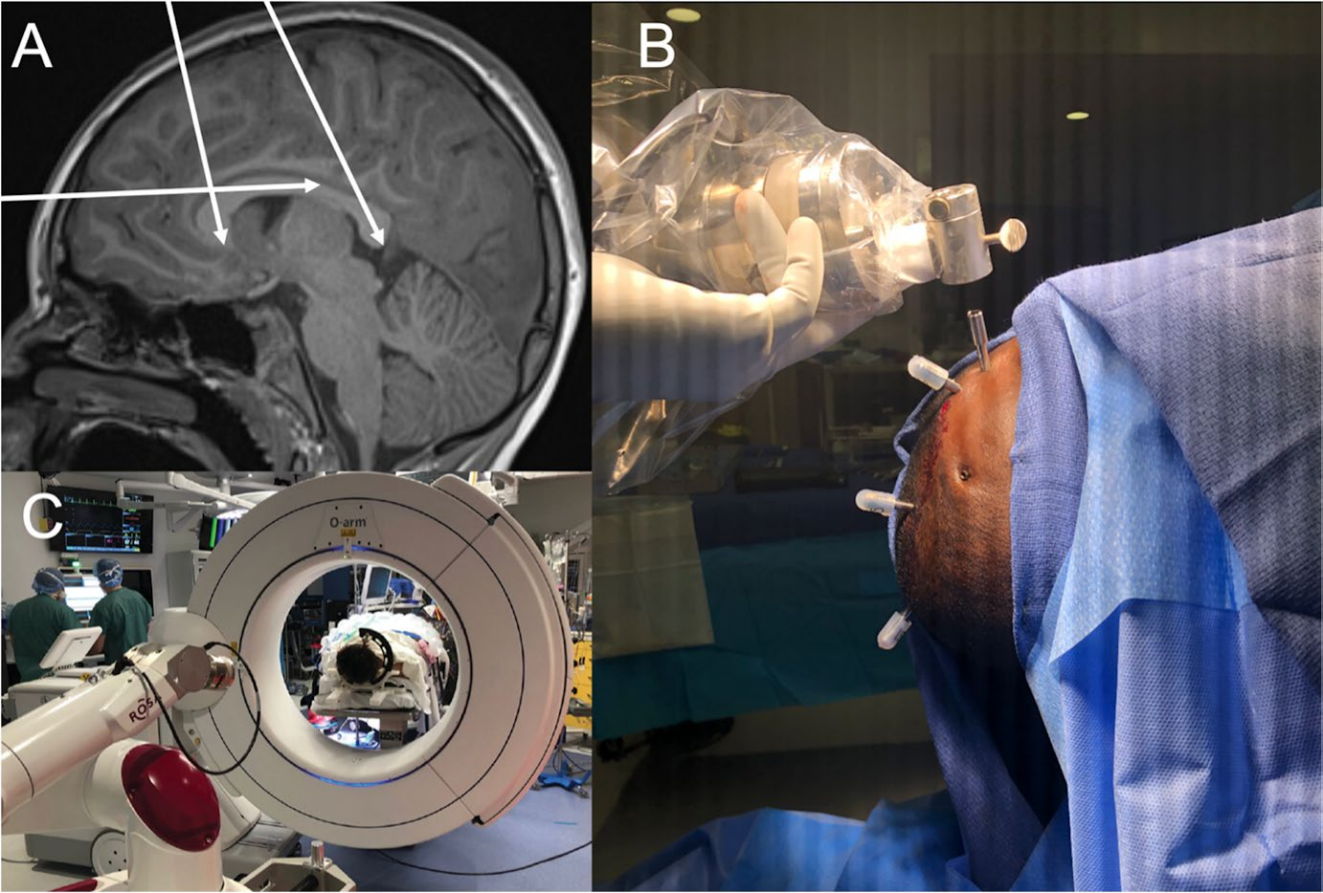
A, Example of a preoperative planning photo using T1‐weighted MRI to visualize LITT catheter trajectory to target the genu, isthmus, and splenium of the corpus callosum. B, Example of capped intraoperative electrode catheters. C, ROSA robot and O‐arm intraoperative imaging system. Not visualized is the Leksell stereotactic system head frame, which is preferred for LITT callosotomy for its flexibility
